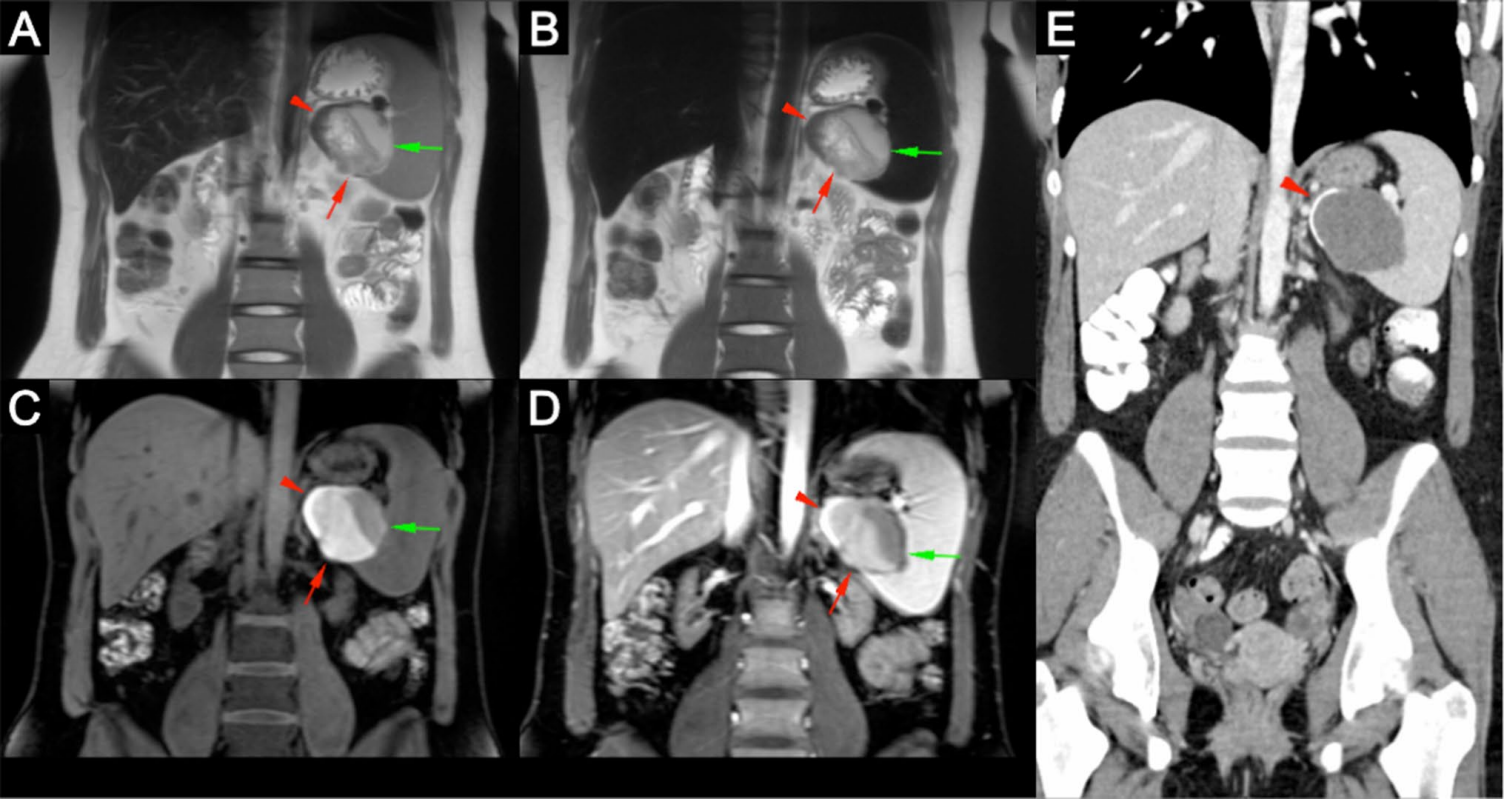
20 year-old female with an indeterminate splenic mass on ultrasound and contrast-enhanced CT suspicious for malignancy. Pre- and post-contrast T2 (a and b) and T1 (c and d) 3.0 T ferumoxytol-enhanced MRI acquisitions show a large splenic lesion with a cystic component (green arrows), and solid component (red arrows) with heterogenous T2 intensity and T1 hyperintensity without contrast enhancement suggestive of proteinaceous content. The solid component also has a linear rim (red arrowhead) of T2 hypointensity and T1 hyperintensity suggestive of a calcification. The prior contrast-enhanced CT study (e) confirms absence of contrast enhancement in the entirety of the lesion and a hyperdense rim (red arrowhead) suggestive of calcification. Altogether the constellation of findings was suggestive of a hemorrhagic cyst which was later confirmed on biopsy
